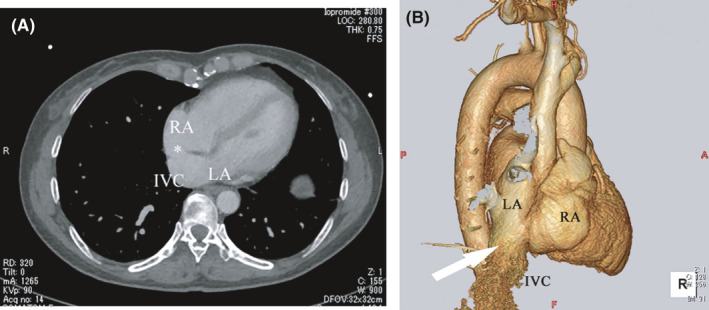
Preoperative contrast‐enhanced computed tomography images. (A) The axial view displays residual atrial septal defects (asterisk) with the inferior vena cava being directed to the left atrium (arrow). (B) The three‐dimensional view demonstrates a direct connection of the inferior vena cava and the left atrium (arrow). IVC, inferior vena cava; LA, left atrium; RA, right atrium
